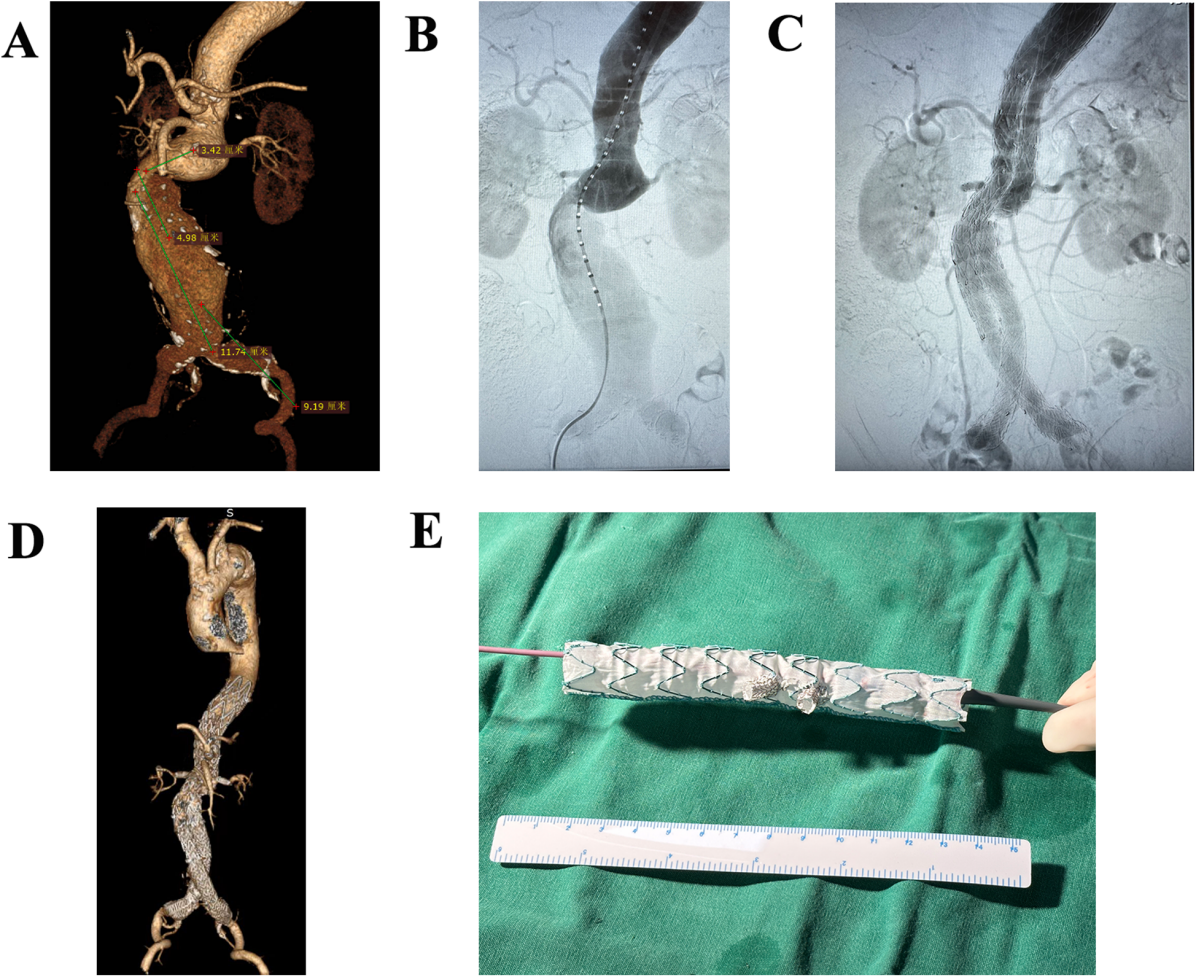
Preoperative and postoperative CTA images of a patient who underwent EVAR. (A) Preoperative CTA image of thoracoabdominal aortic aneurysms. (B) Intraoperative CTA image. (C) Final postoperative CTA image. (D) Follow-up CTA demonstrates satisfactory aortic remodeling without endoleaks. (E) A picture of BSG.
